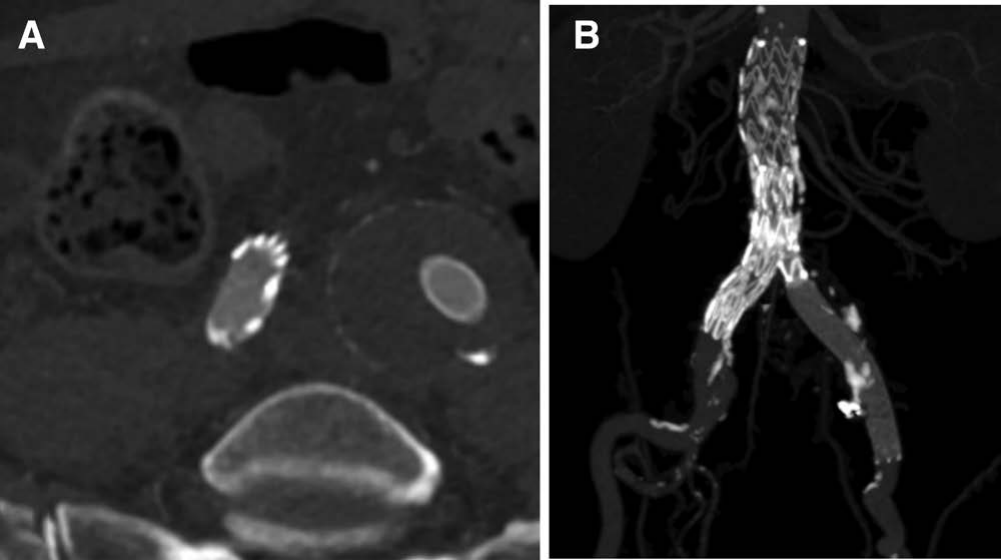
Follow-up computed tomography image after a 1-month anticoagulation with warfarin (target INR 2–2.5) shows (A) complete resolution of the thrombosis in the right common iliac artery and (B) no evidence of endoleak on maximum intensity projection with 3-dimensional reconstruction.
